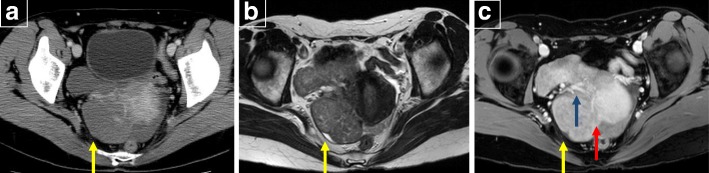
a CT showed the other mass (yellow arrow) in the pelvis. b T2-weighted MRI showed the tumor (yellow arrow) around the uterus. c Enhanced fat suppression T1-weighted image showing the presence of the ovarian vein (gray arrow) in this mass. The boundary between the myoma and the surrounding tissue (red arrow) was partially unclear
